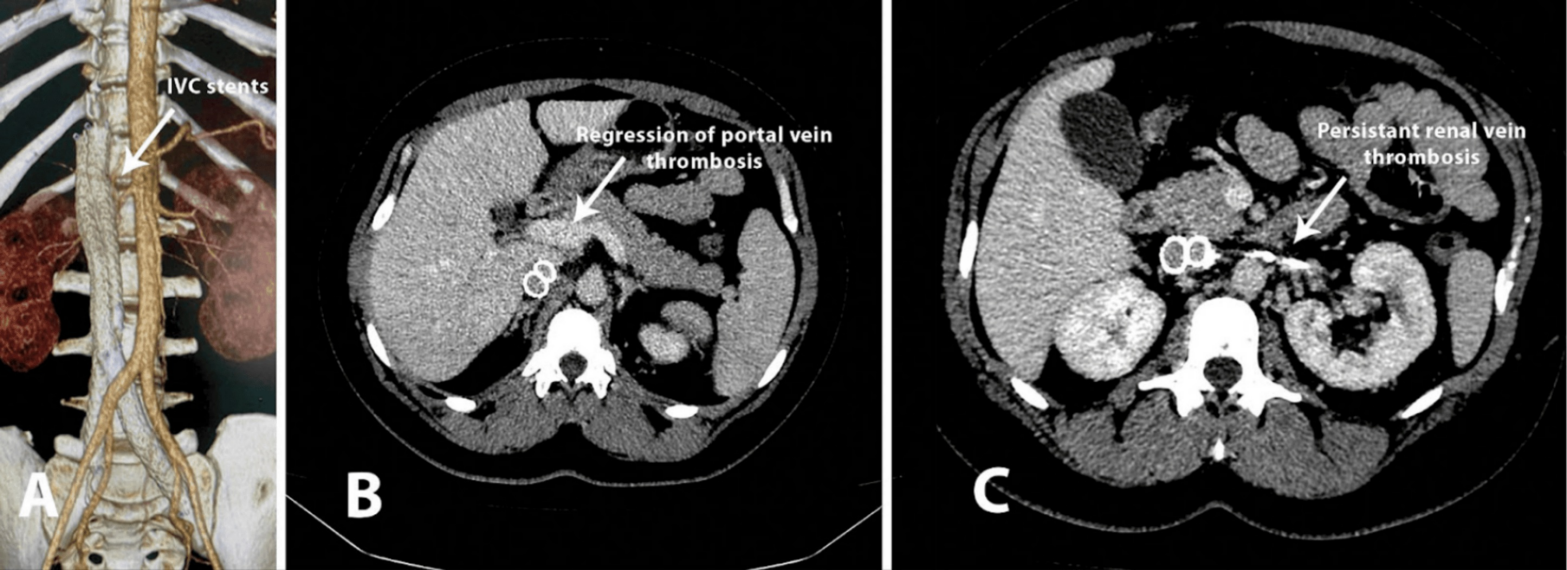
Postoperative CT (A) 3D reconstruction of the patent ilio-caval stents. (B) Regression of the thrombosis in the portal vein. (C) Persistent occlusion of the renal veins IVC: inferior vena cava
